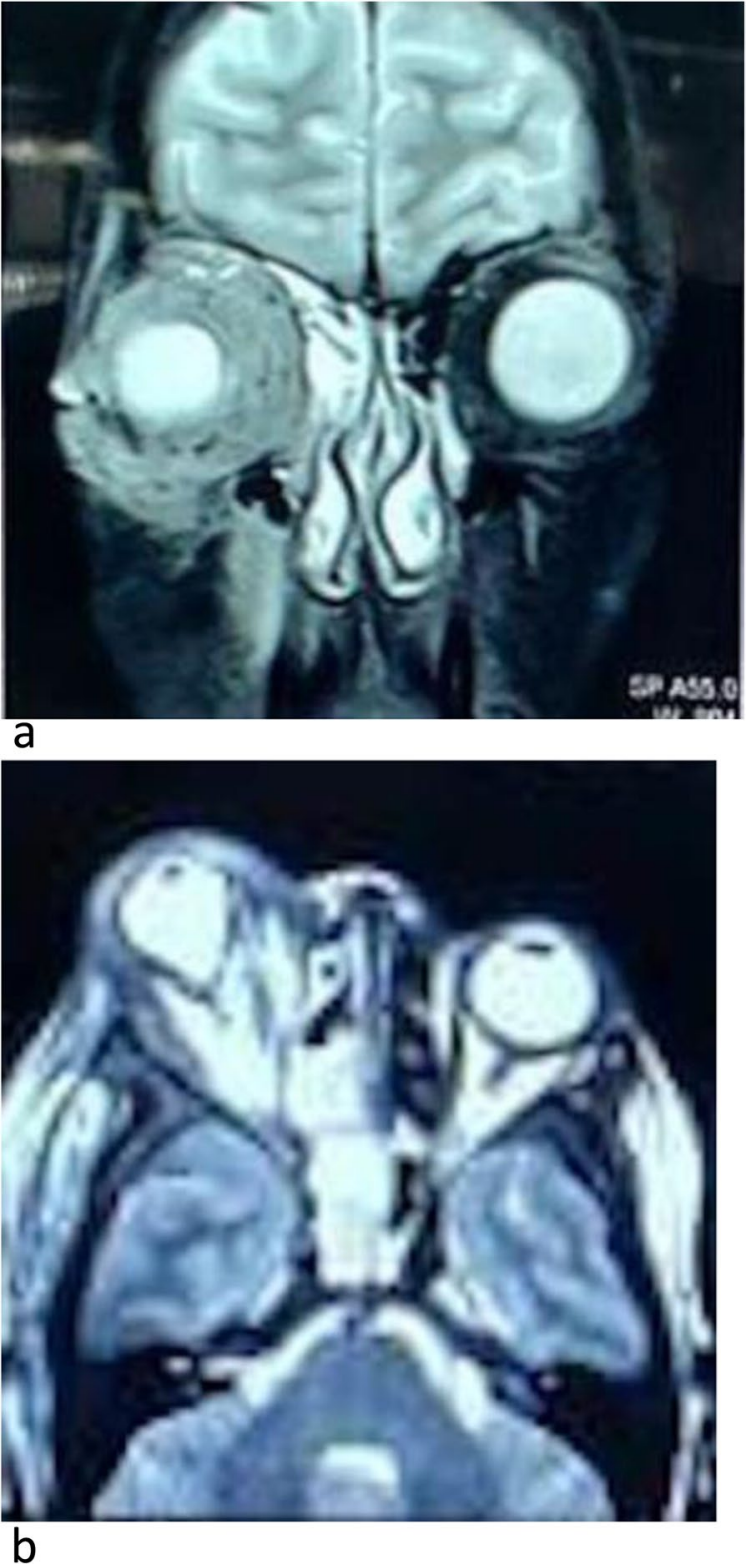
a,b MRI T2W Flair shows hyperintense soft tissue mucosal thickening with peripheral enhancement seen in right maxillary sinuses, right ethmoid air cell, bilateral sphenoid sinus and right frontal with right orbital and periorbital cellulitis
